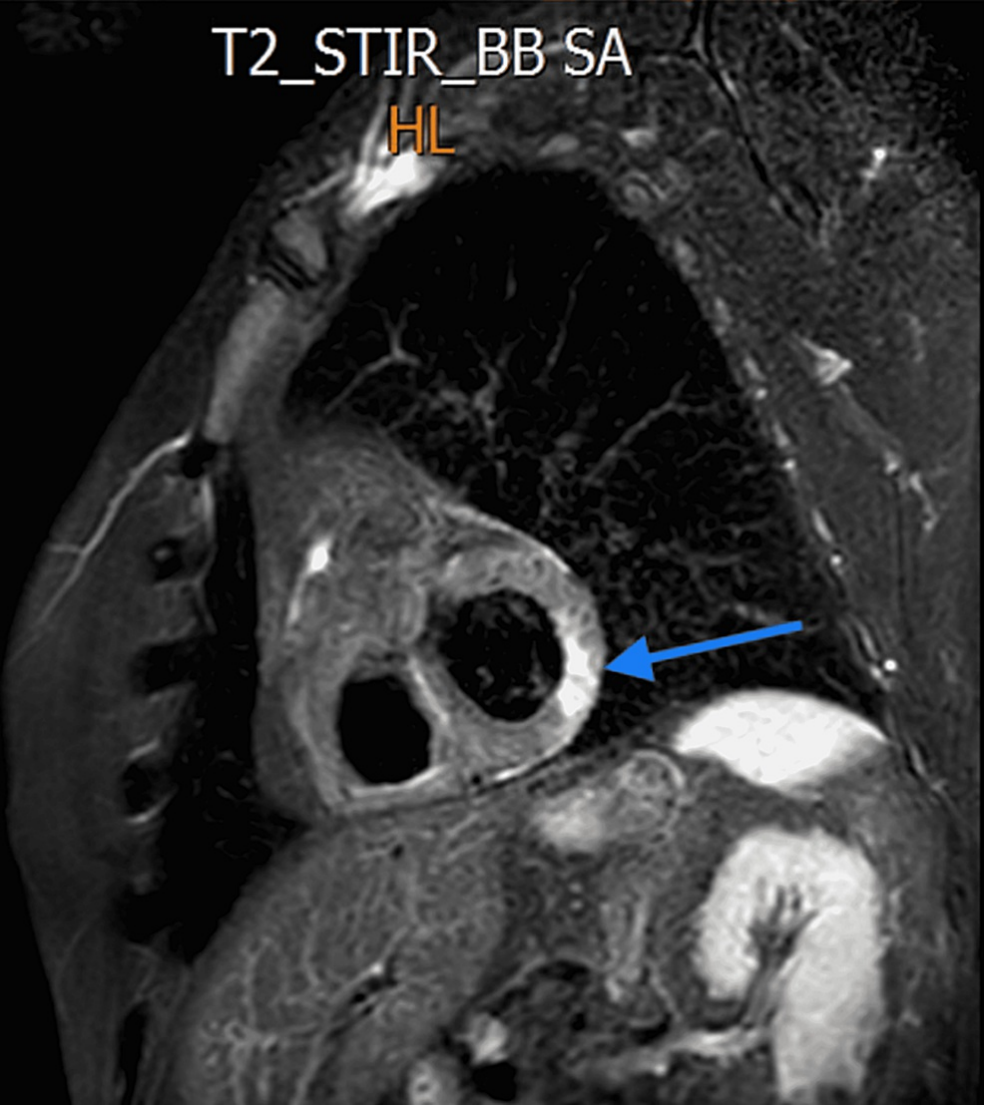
MRI late gadolinium enhancement uptake showing mid myocardial scar in inferolateral wall (blue arrow).
